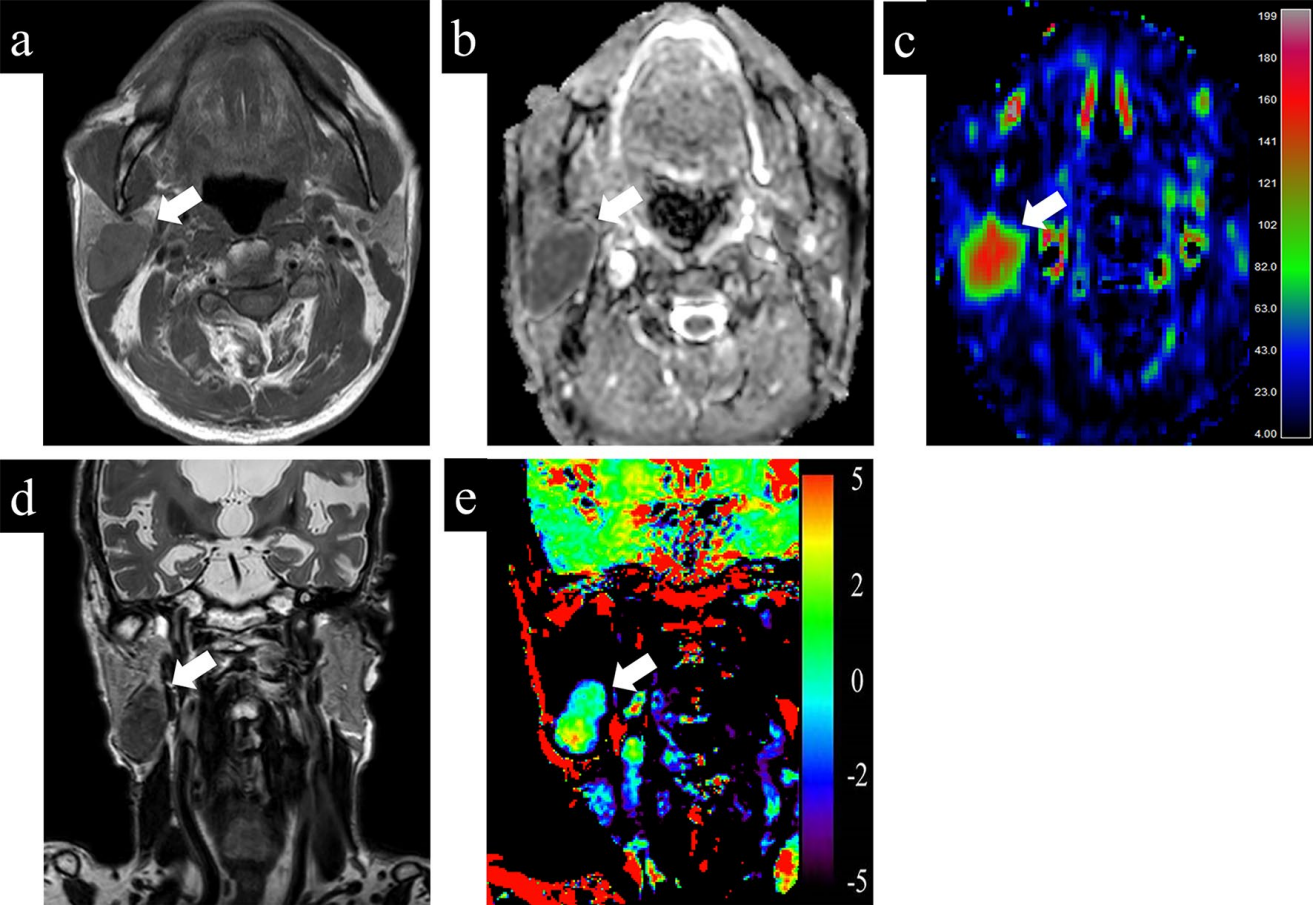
A 79-year-old male with Warthin tumor. (a) T1-weighted image shows a homogeneous low intensity lesion in the right parotid gland. Apparent diffusion coefficient (ADC) (b) and tumor blood flow (TBF) (c) maps show the lesion with the mean ADC value of 0.85 × 10^−3^ mm^2^/s and the mean TBF value of 133.06 mL/100 g/min, respectively. On T2-weighted coronal image (d), the lesion shows lower signal intensity than the adjacent parotid gland parenchyma. Amide proton transfer (APT) (e) map shows the lesion with the mean APT-related signal intensity of 1.24%. An arrow in each image indicates the lesion.
